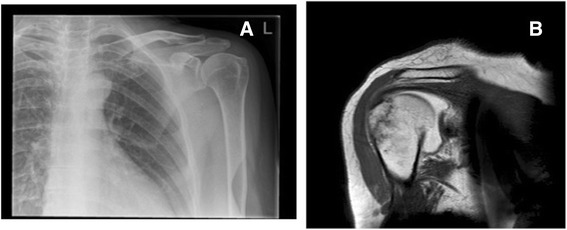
a Left shoulder Plain radiography coronal plane which was reported as normal and (b) the subsequent MRA on the same patient (Left shoulder) which revealed an occult fracture of the greater tuberosity
